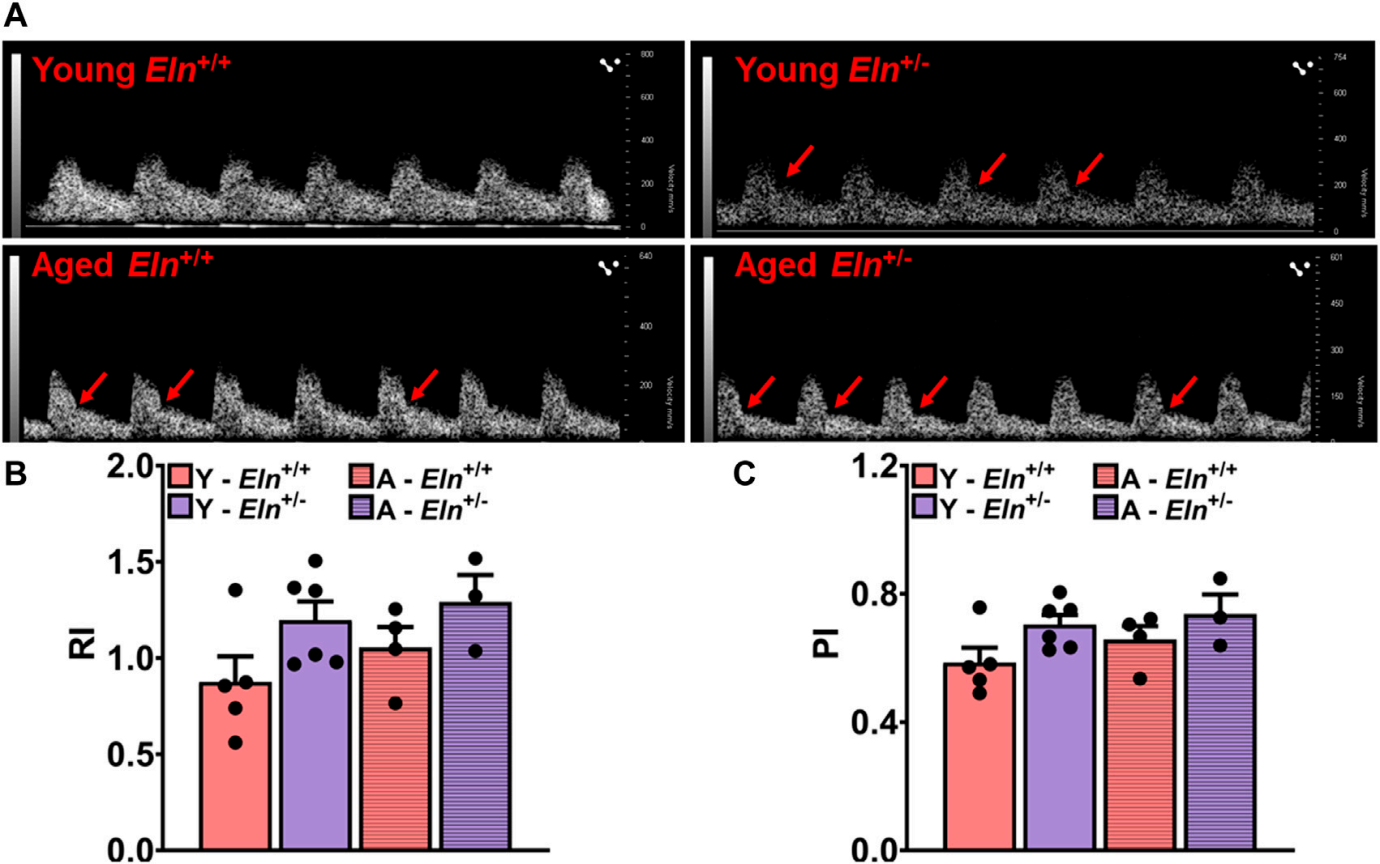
Doppler ultrasonography of renal arteries in anesthetized young and aged *Eln*
